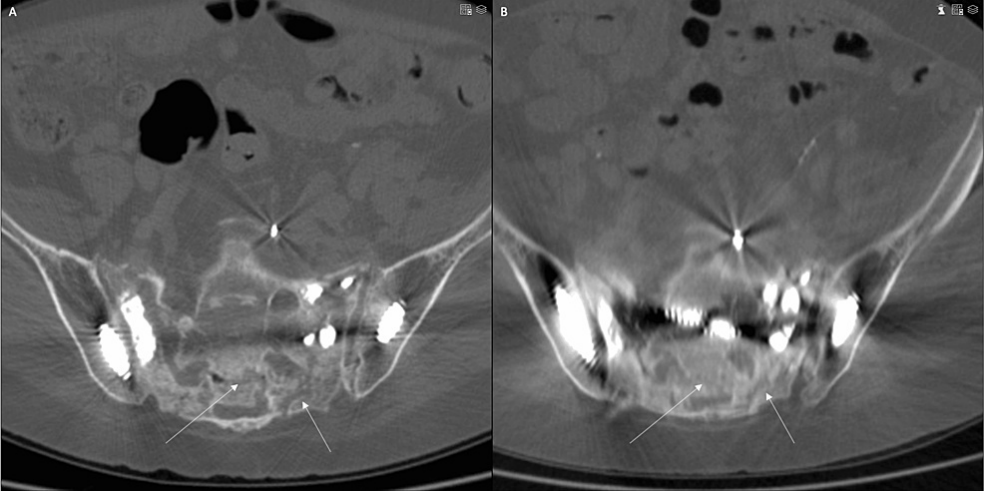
Axial computed tomography of the pelvis before (A) and 10 months after (B) iliosacral fixation and sacroiliac joint fusion. Partial consolidation of the U-shaped fracture at 10 months postoperative scan is visible (white arrows).
